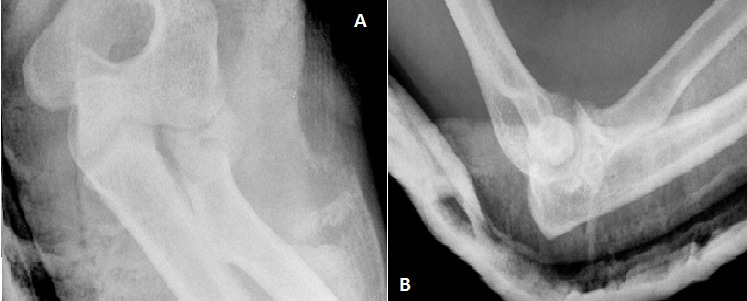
Radiographie du coude droit de face et de profil: facture comminutive de la tête radiale Mason III (A,B)
